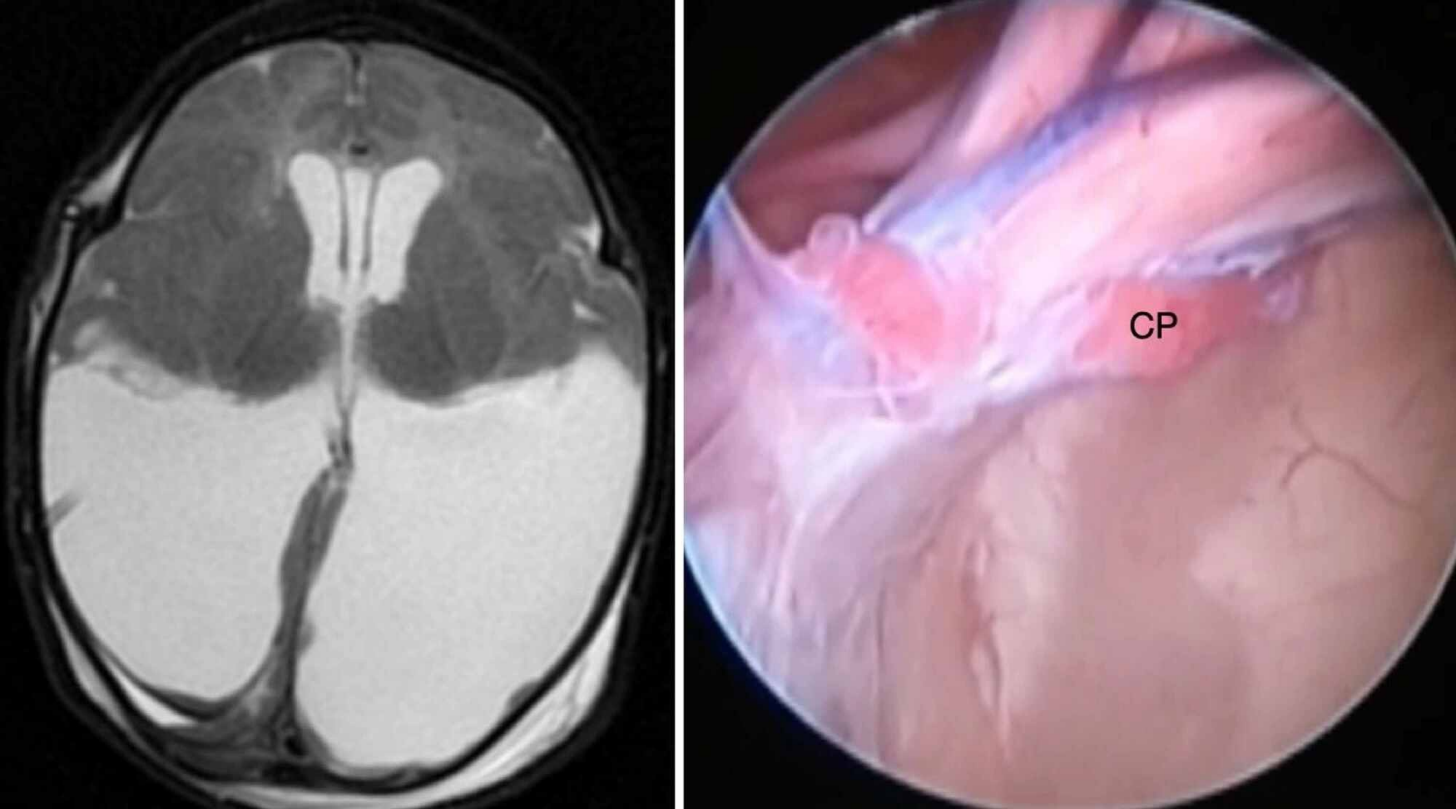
The type V anatomic variation of the glomus of choroid plexus (GCP). Type V GCP is classified as the absence of GCP. On both sides, the GCP is not visible in an axial view on T2-weighted magnetic resonance imaging (left). In this endoscopic view of the right lateral ventricle, the choroid plexus (CP) has the same appearance throughout the lateral ventricle with no glomus formation.
